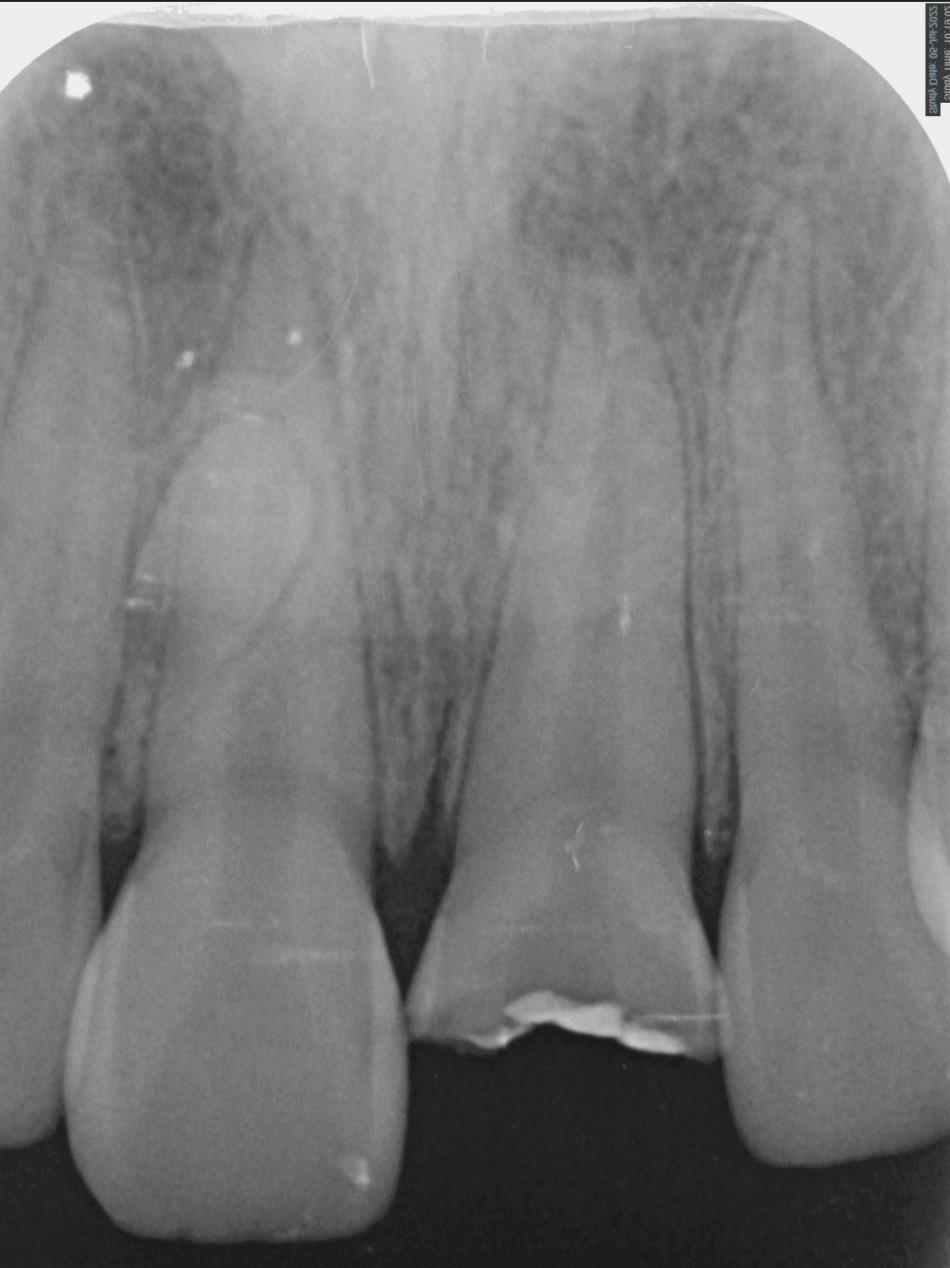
Periapical radiograph showing the fractured maxillary left central incisor
